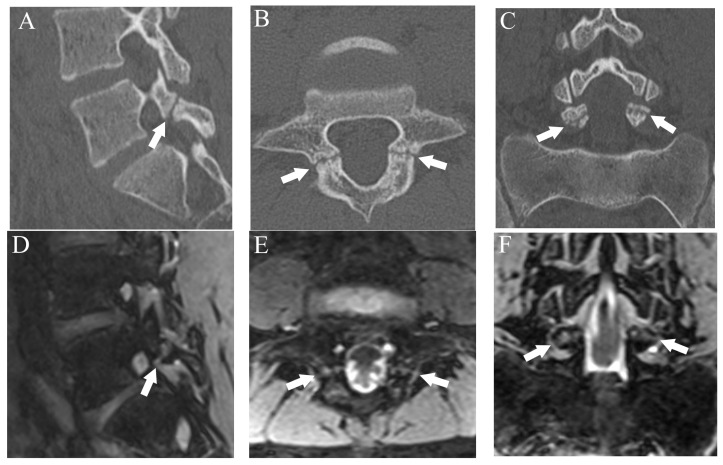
Comparison of bilateral grade 3 fractures at L5 from an 11-year-old male in multiple planes between CT and DESS. (A–C) CT shows complete fractures in L5 pars interarticularis on the right-sided sagittal, axial, and coronal plane, respectively (arrows). (D–F) DESS shows comparable complete fracture on the right-sided sagittal, axial, and coronal plane, respectively (arrows).
